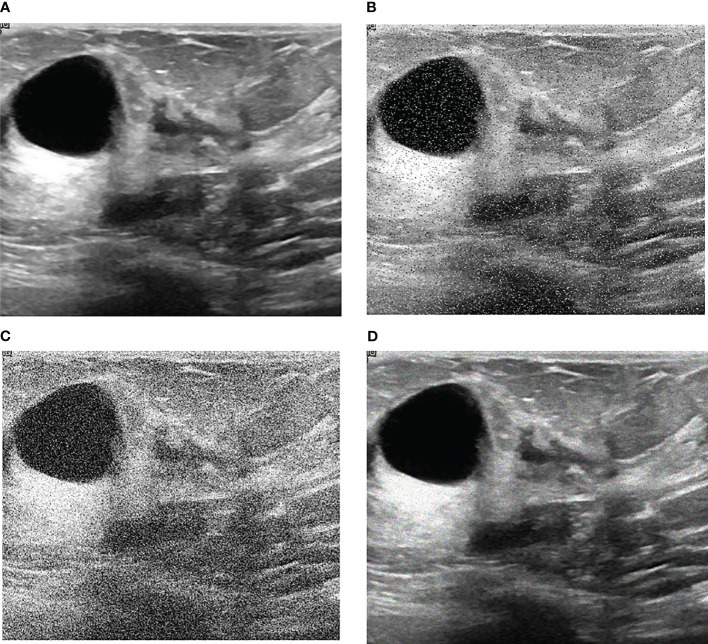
Noise impact on breast ultrasound images. (A) Original Image. (B) Salt & Pepper Noise. (C) Gaussian Noise. (D) Speckle Noise.
